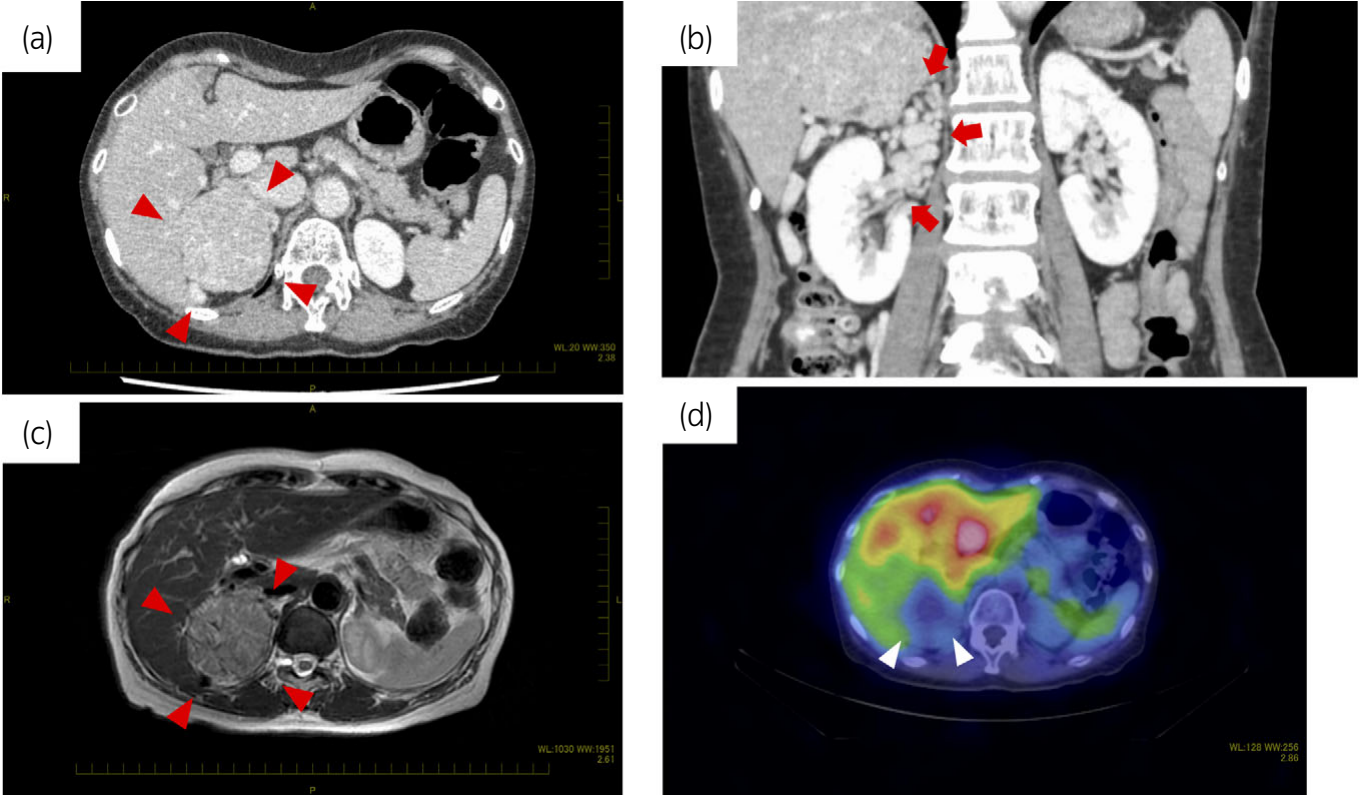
CT scan showed (a) a well‐circumscribed tumor at the superior pole of the right kidney and (b) well‐developed blood vessels around the lesion. (c) The tumor showed a high signal on T2‐weighted MRI, and there was no invasion. (d) ^123^I‐MIBG scintigraphy was negative for radionuclide accumulation in the mass.
